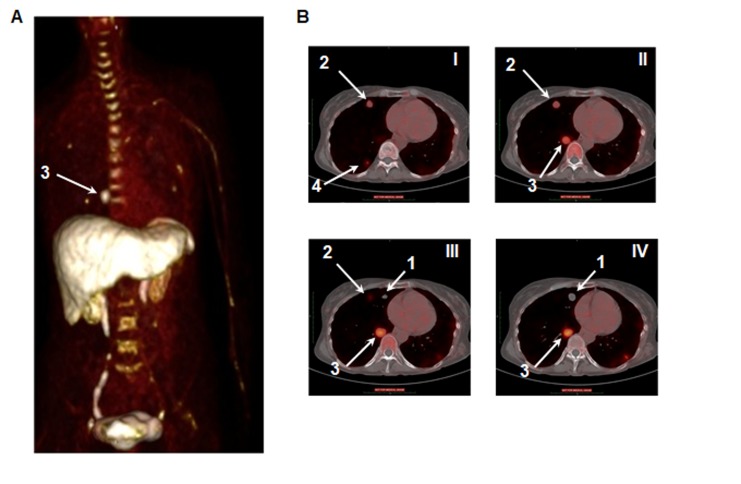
^64^Cu-plerixafor identifies pulmonary metastases of ACC A. PET Maximum Intensity Projection (MIP) of a patient with metastatic ACC 40 minutes following injection of ^64^Cu-plerixafor. B. Thoracic transaxial plane images of PET as in A with CT co-registration. Images are rostral (I) to caudal (IV). Right lung nodules, as indicated by the white arrows, were numbered prior to resection to allow for subsequent analyses. Nodule 1 was the only target nodule without focal radiotracer uptake.
